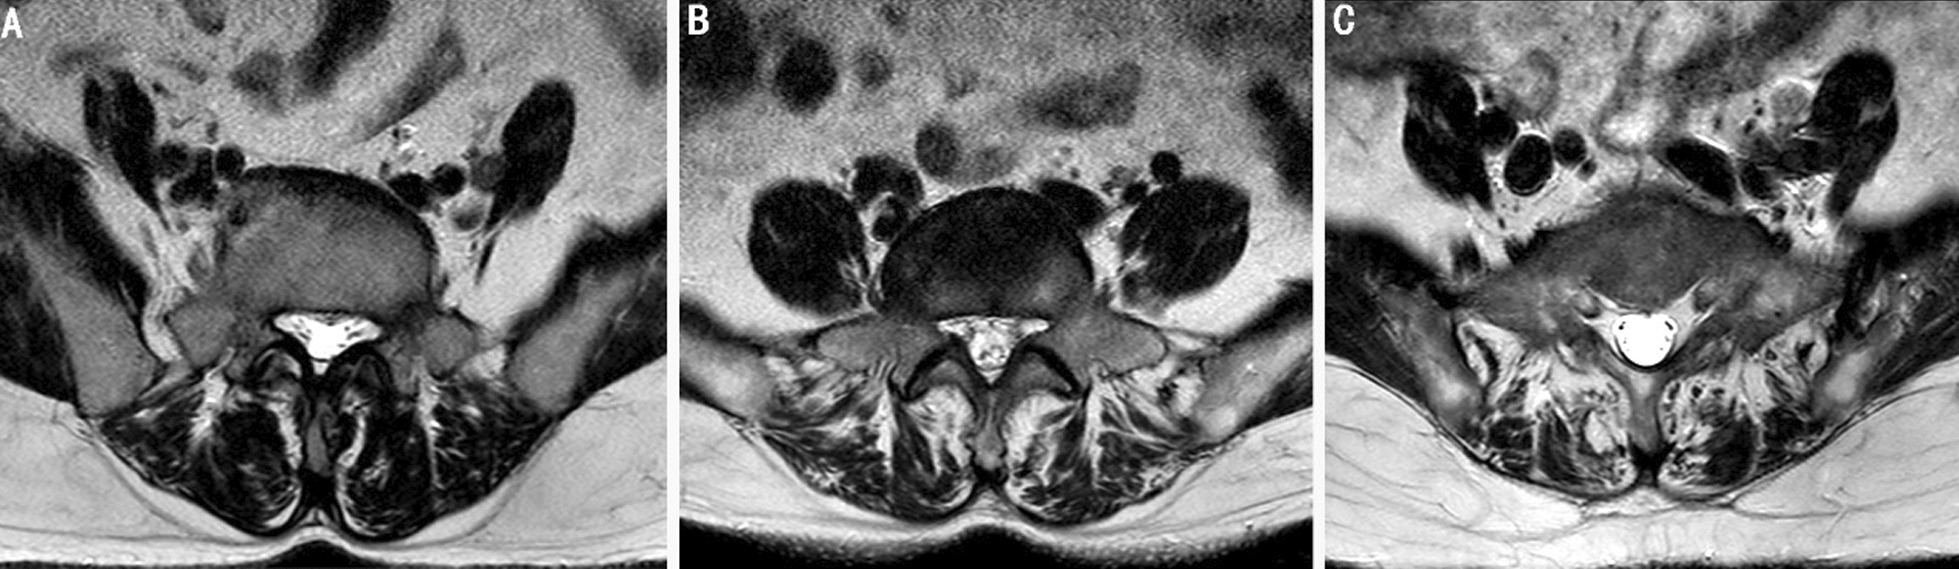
Muscular location and fat infiltration degrees in cross section with T2 axial Magnetic Resonance Imaging (evaluation with simplified 3-grade system) A Grade 1 =  < 10% fat infiltration, B Grade 2 = 10–50% fat infiltration, C Grade 3 =  > 50% fat infiltration
